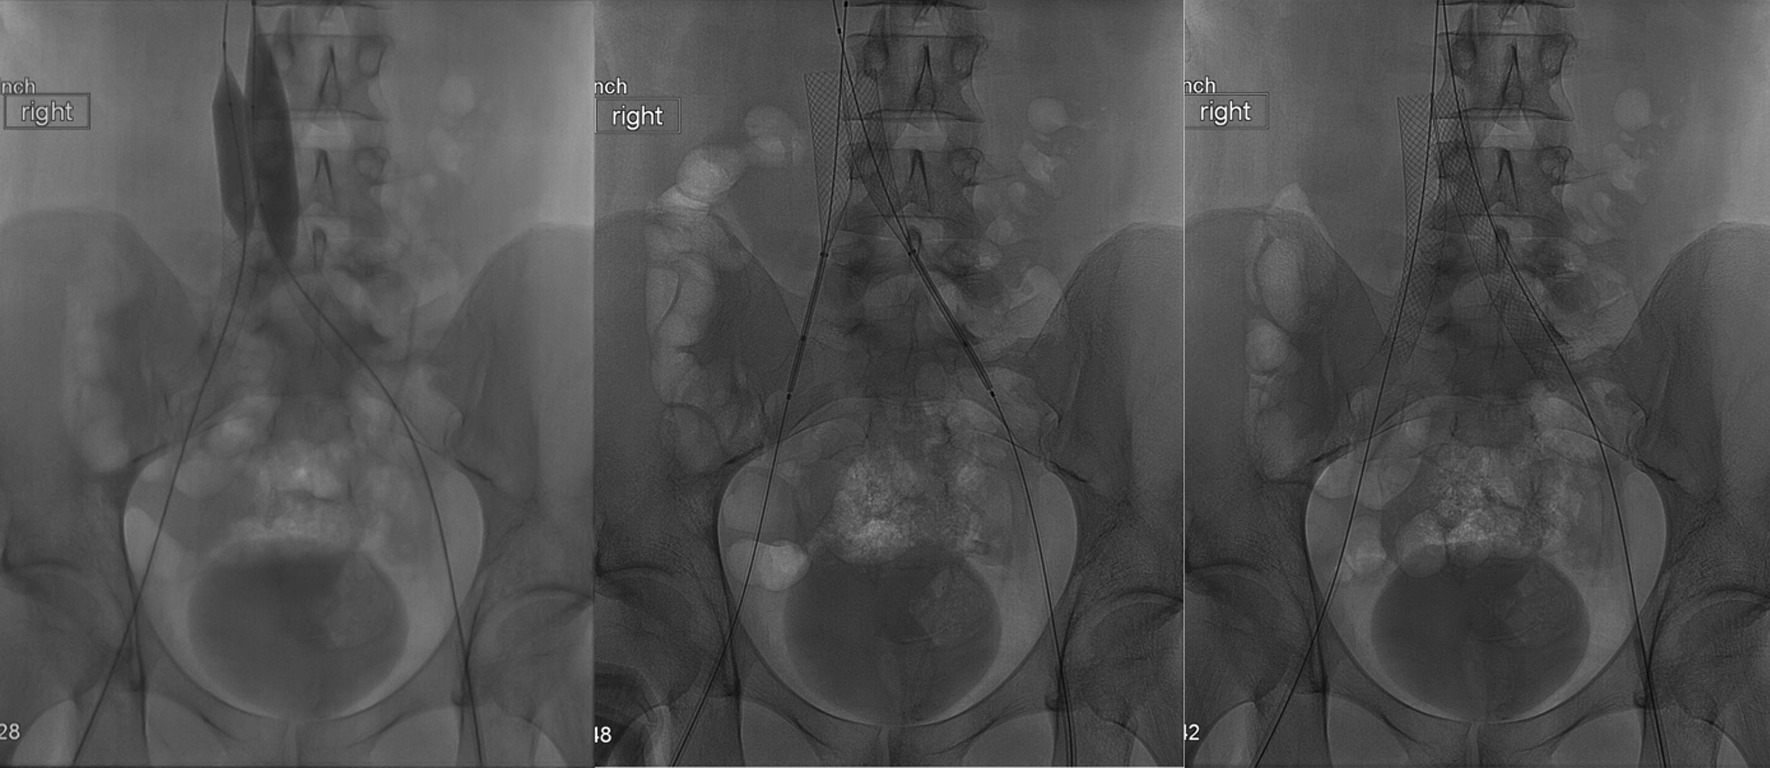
Intraoperative venograms show ballooning (left), stent deployment (middle), and completion of deployment (right). Stents were placed bilaterally after Doppler flow analysis showed diminished blood flow in both left and right internal iliac veins
